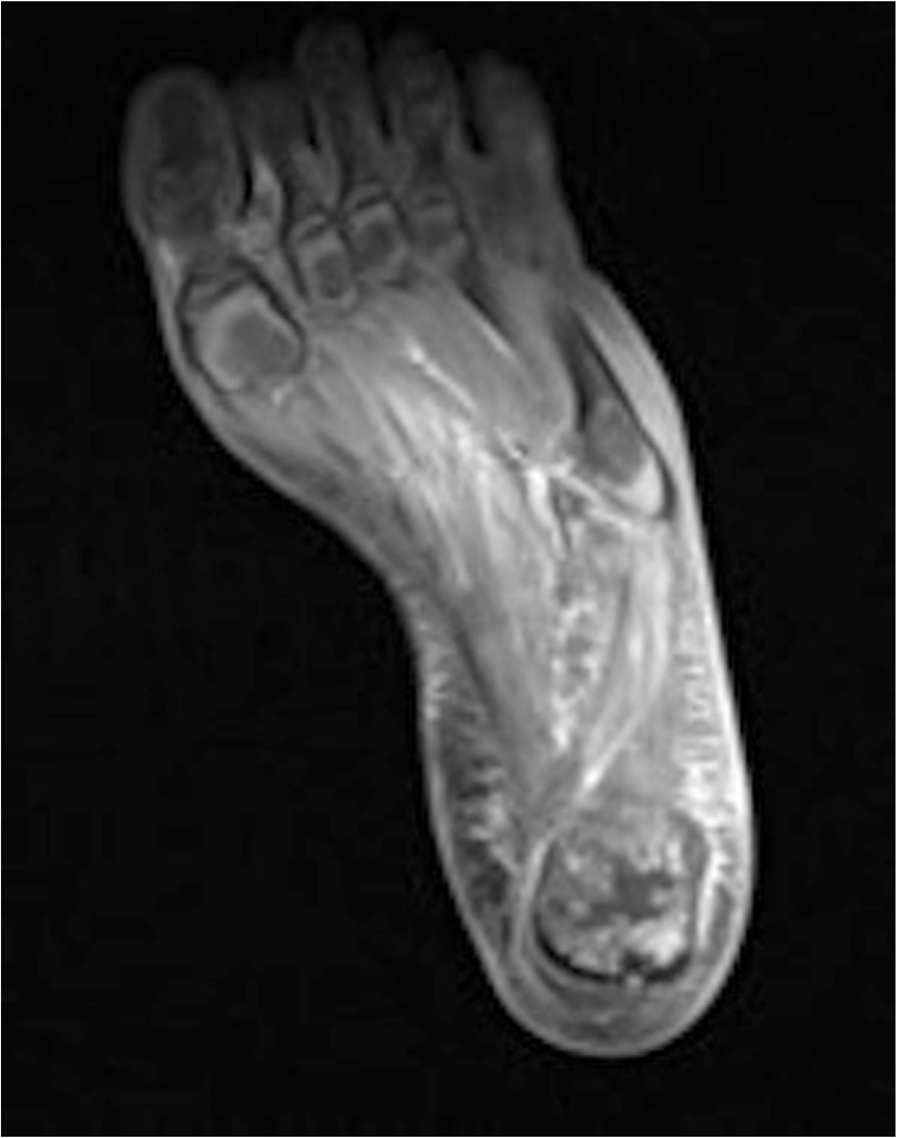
Axial magnetic resonance imaging scan of the left foot with osteomyelitis of the calcaneum
